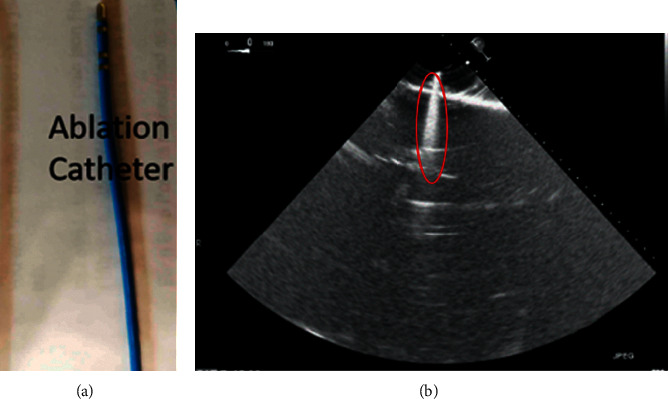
(a) Shelley medical ablation catheter. (b) Corresponding 2-D ultrasound image of (a).
